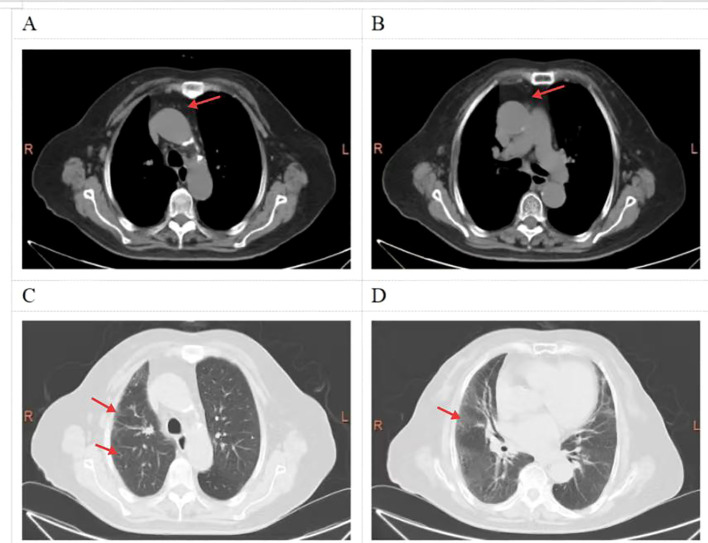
Chest CT scan. (A, B) show no space-occupying lesions in the anterior mediastinum or lungs; (C, D) demonstrate bronchiectasis and interstitial changes in the lungs.
